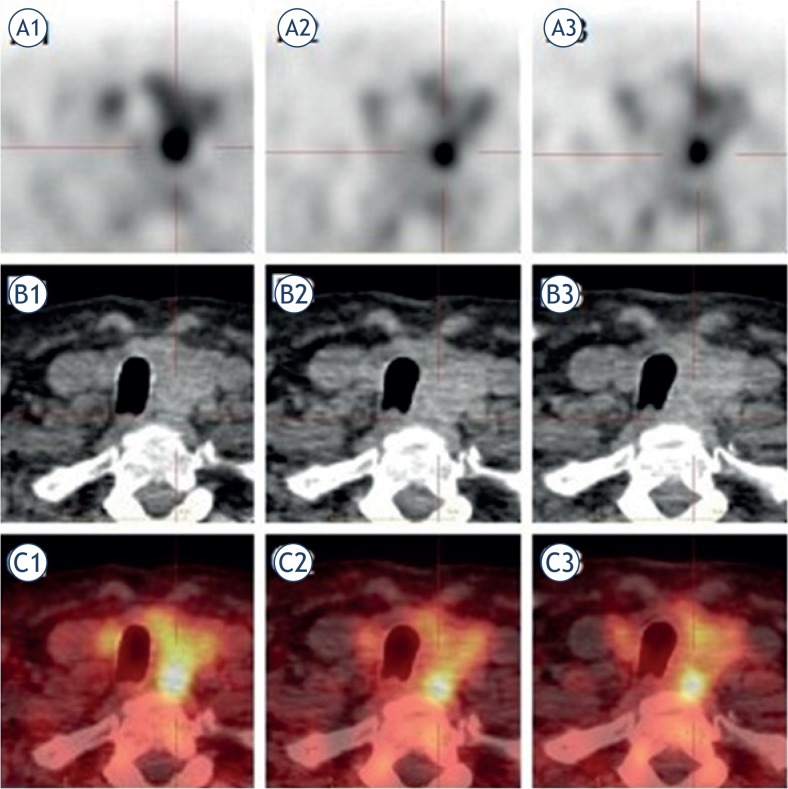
A secluded lower left LREPT. FCH PET/CT was performed in triple-phase after administration of 100 MBq of FCH. The LREPT is well delineated according to the thyroid tissue on PET axial image in the first (A1), the second (A2) and the third phase (A3). SUV_mean_ in the LREPT and the thyroid tissue was in the first phase 6.3 and 3.6, in the second phase 7.1 and 3, and in the third phase 6.6 and 2.9. The CT axial image of the LREPT and the thyroid tissue in all three phases (B1, B2, B3), and the FCH PET/CT axial fusion image in all three phases (C1, C2, C3).
